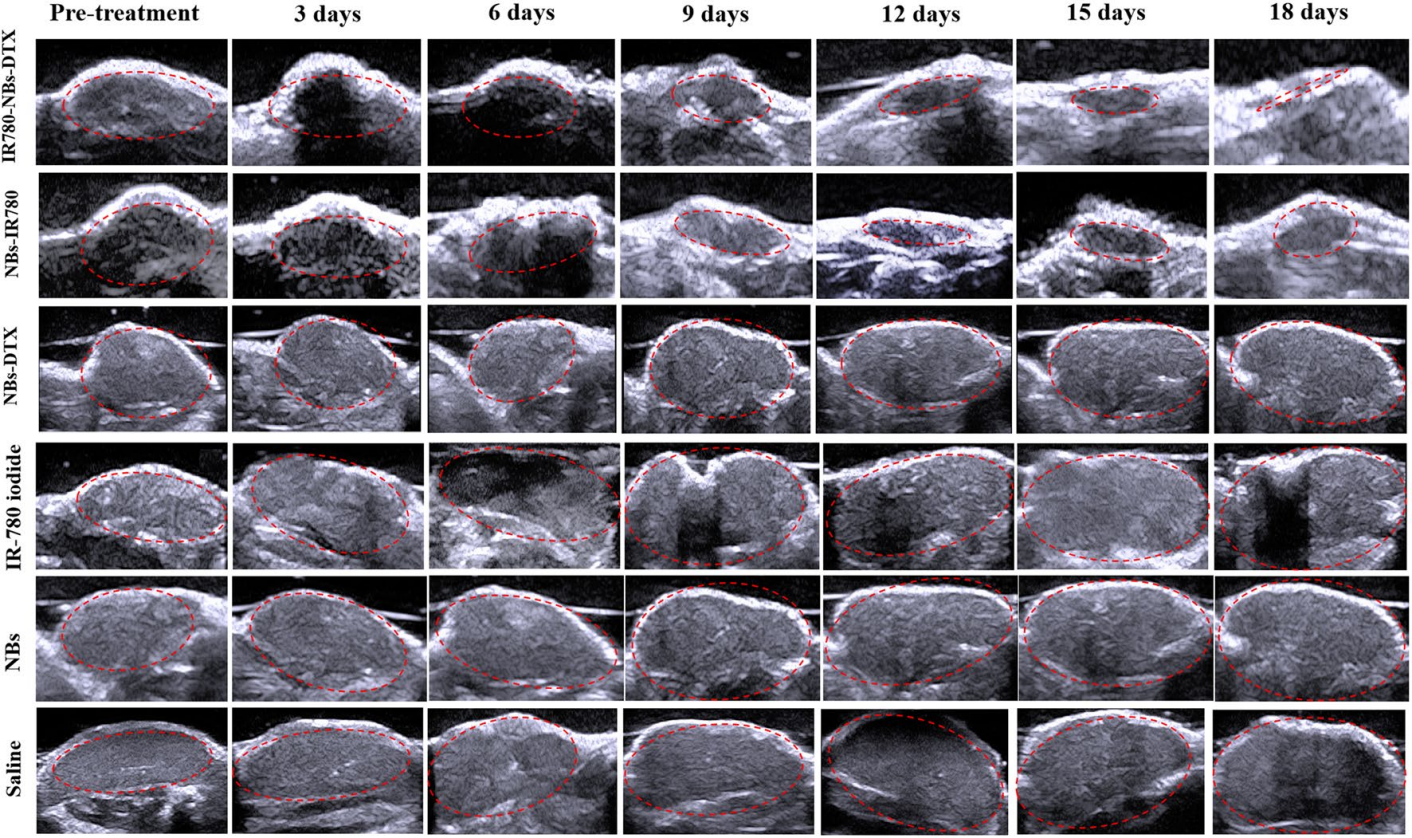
Changes in tumor volume were monitored by traditional ultrasound before and after combined treatment with different contrast agents (IR780-NBs-DTX, NBs-IR780, NBs-DTX, IR-780 iodide, NBs group, and saline). Red dotted lines represent tumors observed by ultrasound imaging.
